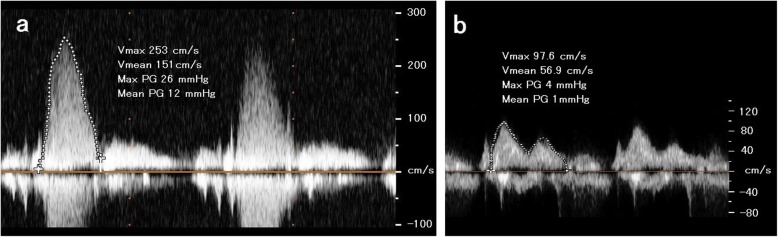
a Transaortic echocardiography pressure peak gradient, measured by continuous wave Doppler across the left ventricular outflow tract before surgery. b Transaortic echocardiography pressure peak gradient measured by continuous wave Doppler across the left ventricular outflow tract after surgery. Vmax, maximum flow velocity; Vmean, mean flow velocity; Max PG, maximum pressure gradient; Mean PG, mean pressure gradient
